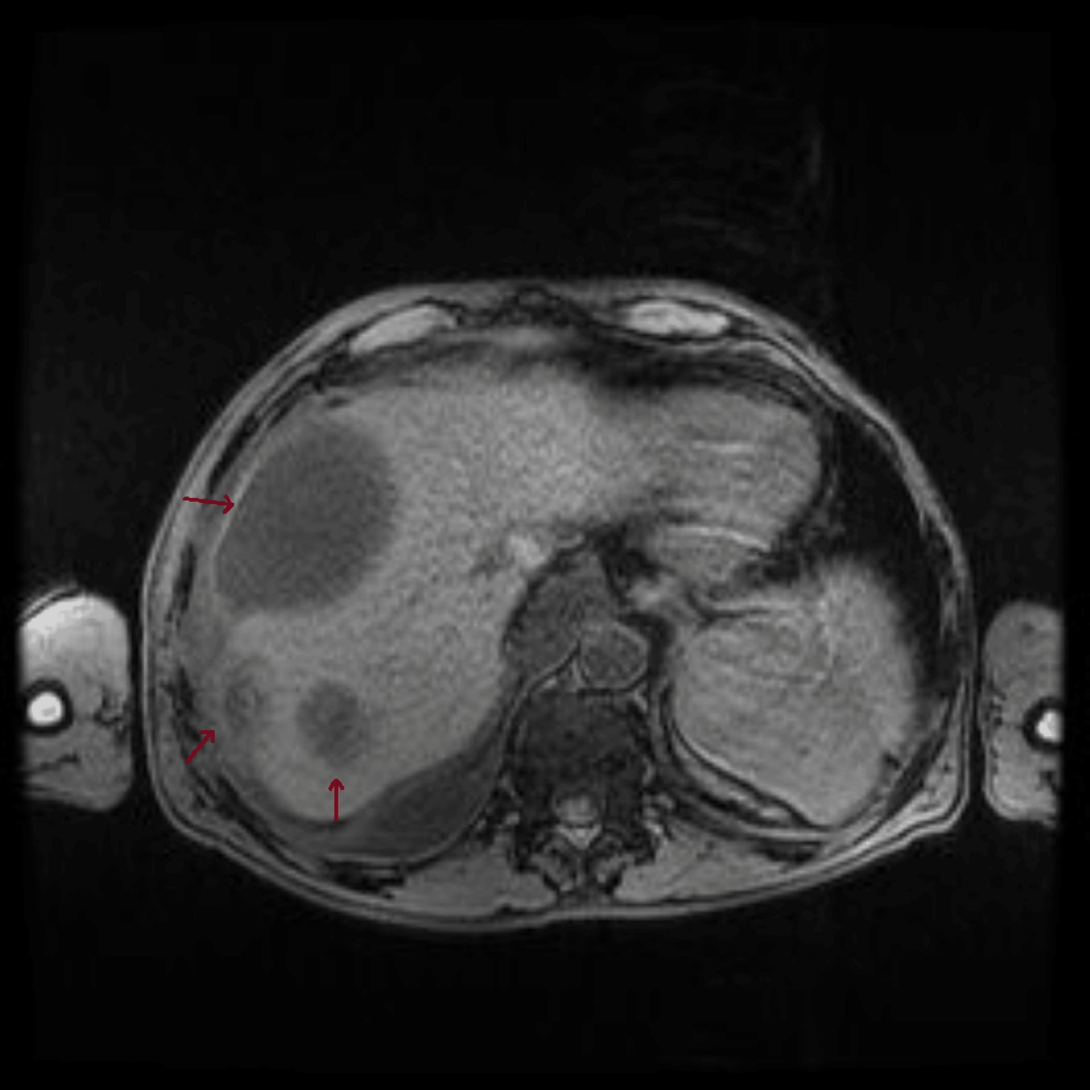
Abdominal MRI performed on the fourth day of hospitalization shows multiple hepatic lesions (arrows), suggestive of hepatic abscesses. The image shows the largest lenticular lesion centered on segment VIII, measuring approximately 10x8 cm.
